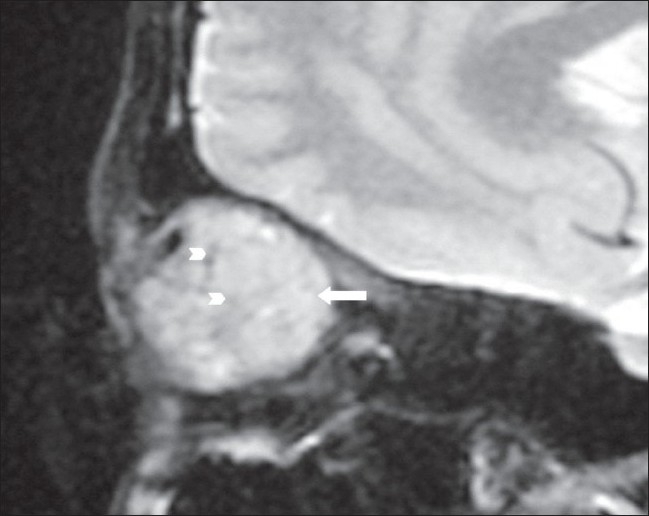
Sagittal T2W MRI image shows a hyperintense lesion (arrow) with signal voids (arrowheads) within
